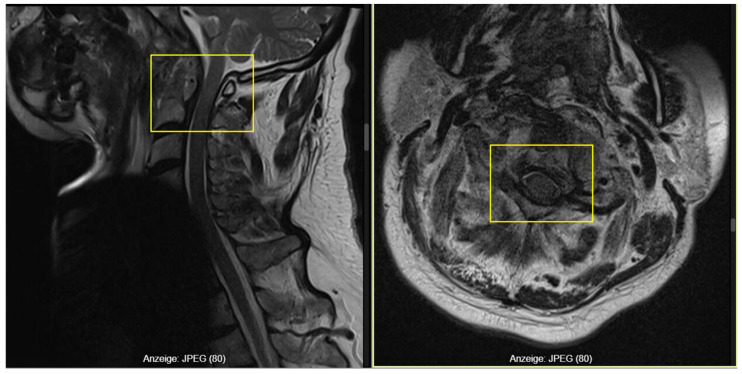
MRI-T2 demonstrating retro-odontoid enhancing lesion (left frame) with a moderate canal stenosis and a preserved CSF rim (right frame).
